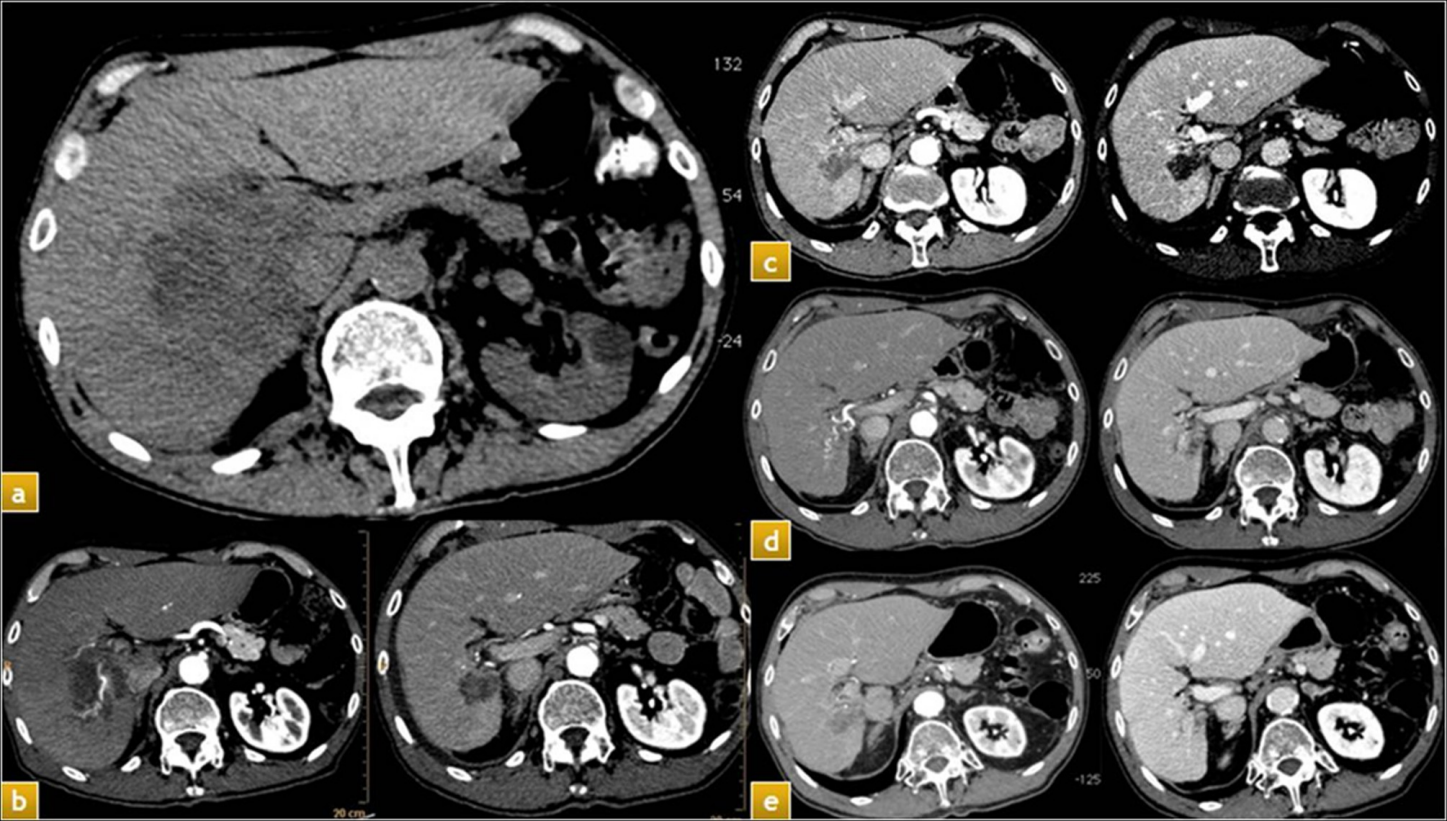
Patient with large HCC in the right lobe with main trunk portal thrombosis: a) baseline CT; b) CT assessment imaging one month after TARE; c) CT imaging six months after TARE; d) CT imaging 12 months after TARE; e) CT imaging 24 months after TARE.
